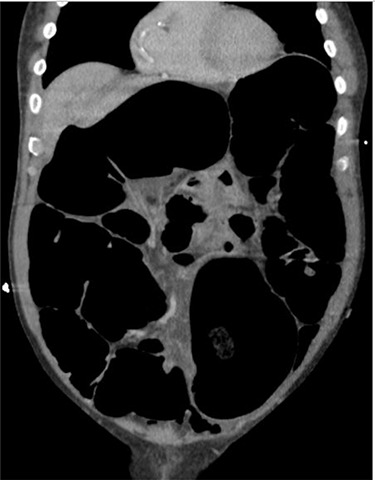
Case one: coronal slice of a CT scan of the abdomen and pelvis with intravenous contrast showing dilated large bowel.
